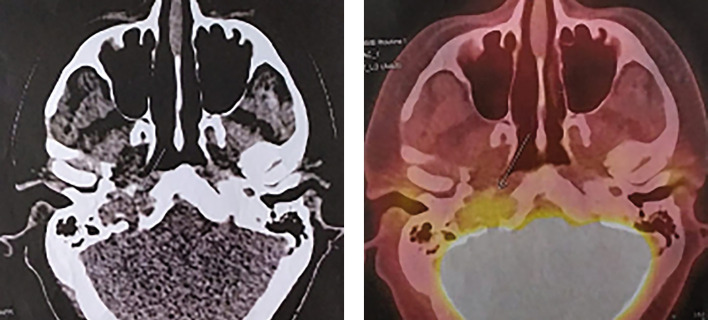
Рисунок 2. На серии ПЭТ/КТ выявляются очаги патологического накопления 18F-ФДГ: в большом крыле клиновидной кости справа с макс SUV 4,85, по КТ — мягкотканное образование, деструирующее клиновидную кость и прилегающие участки ската и пирамиды правой височной кости с образованием внекостного компонента, распространяющегося интракраниально и дистально по ходу внутренней сонной артерии до уровня С1, размерами около 2,8х1,5 см (указано стрелкой).
